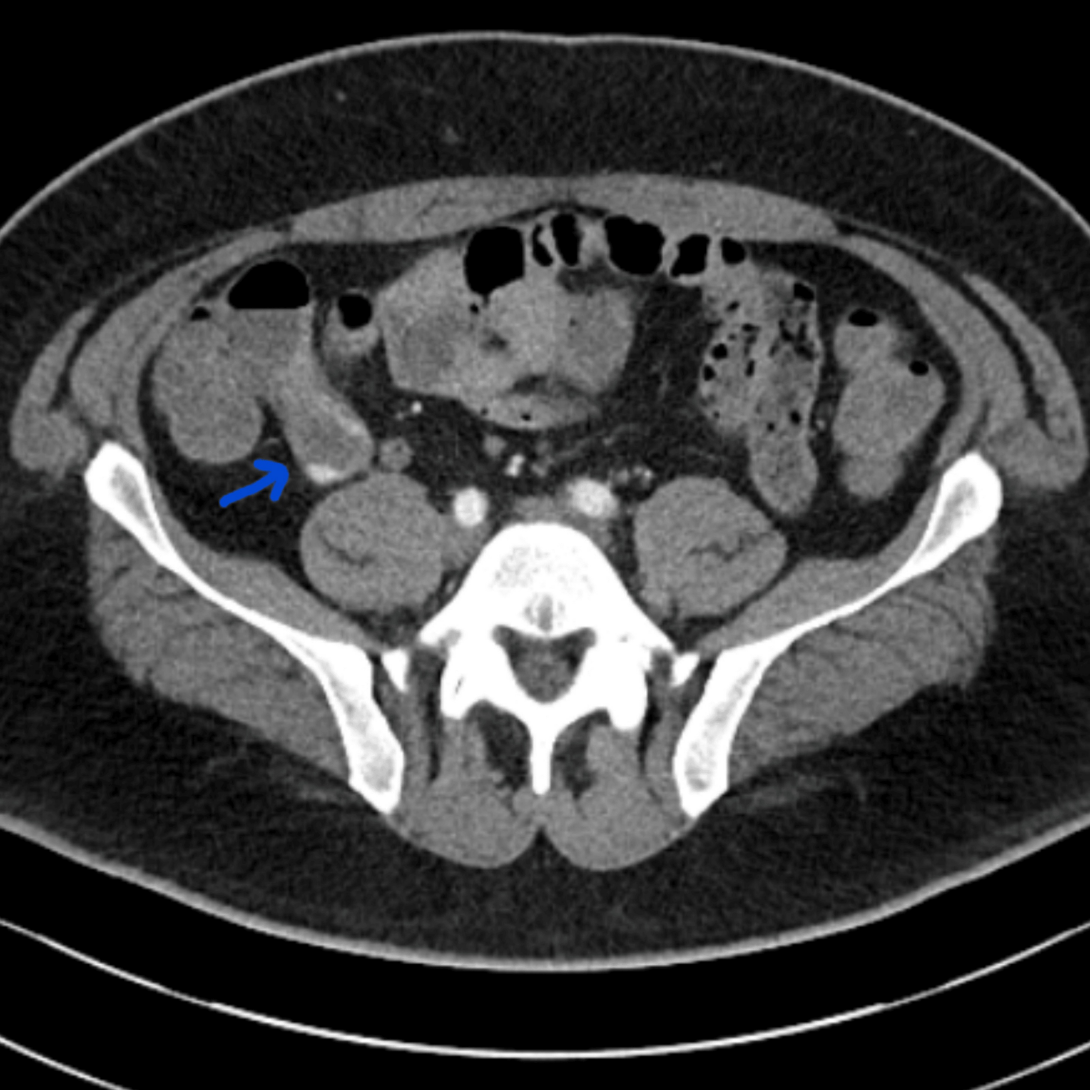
Arterial-phase CT angiogram showing focal contrast extravasation within the thickened appendix, indicative of active bleeding. CT, computed tomography
